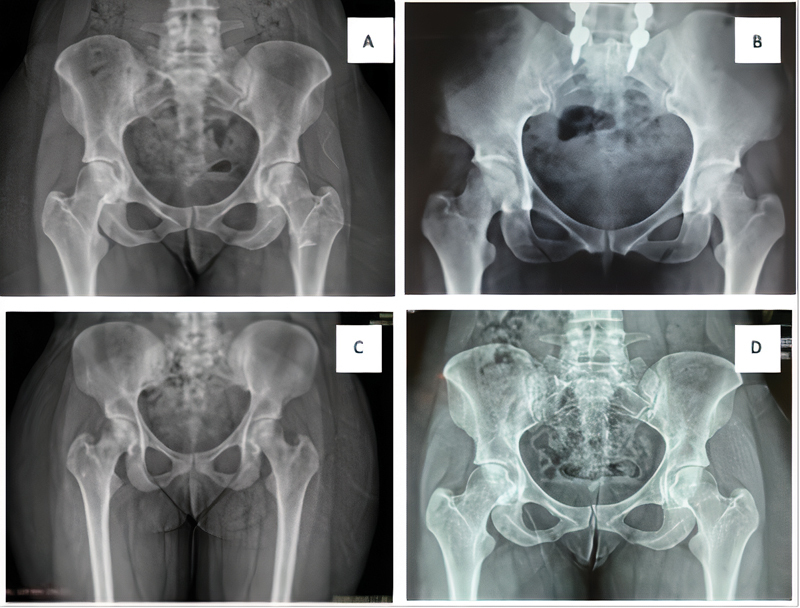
Preoperative radiographs of patients 1 (
